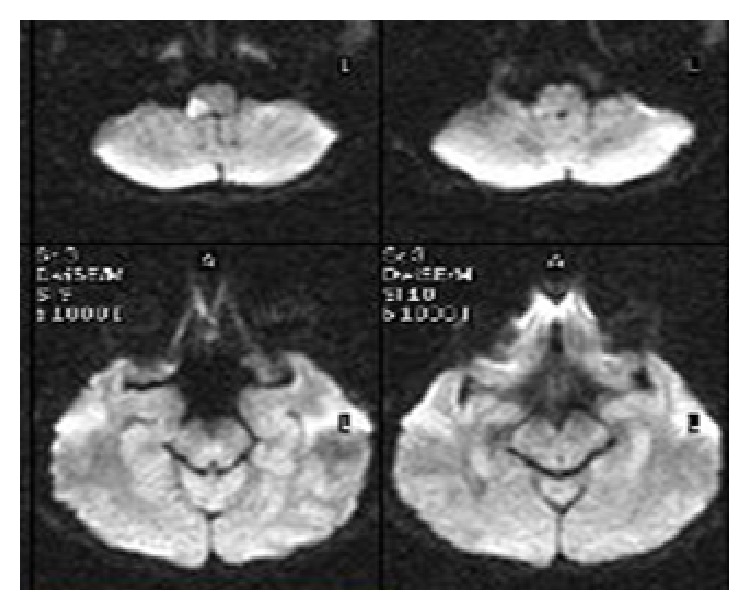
The diffusion restriction which led to the acute infarct, holding the posterolateral bulbus right hemisphere according to the results of the diffusion MRI.
